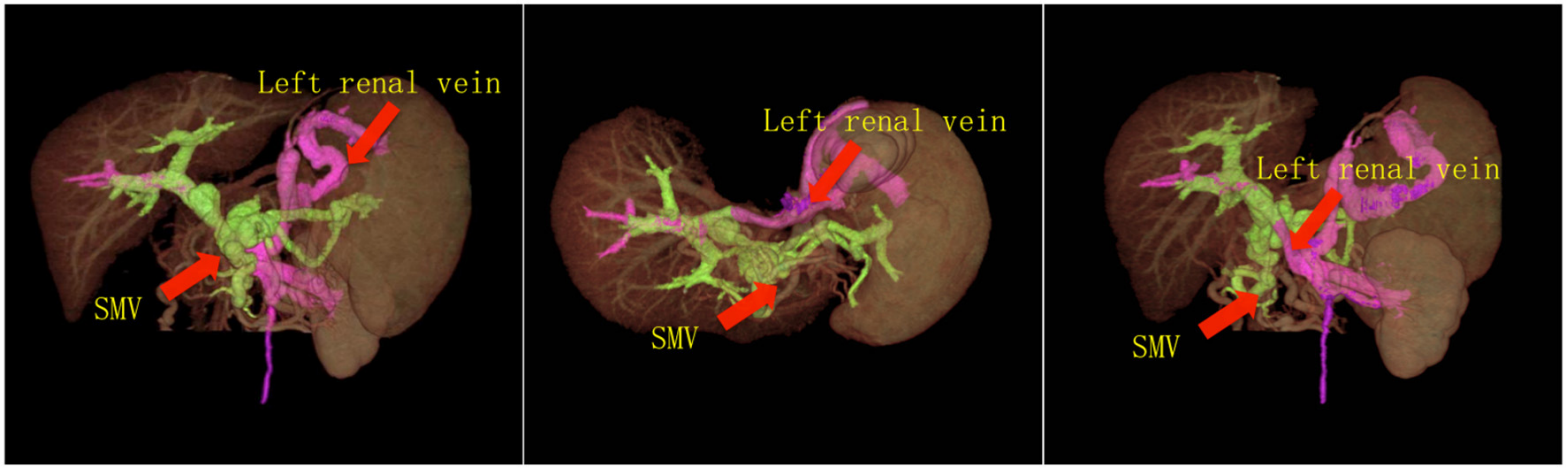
3-D CT images reconstruction of the portal system. The CT images showed that the portal vein perfusion was composed of blood flow from both PV and left renal vein, without regional portal hypertension.
